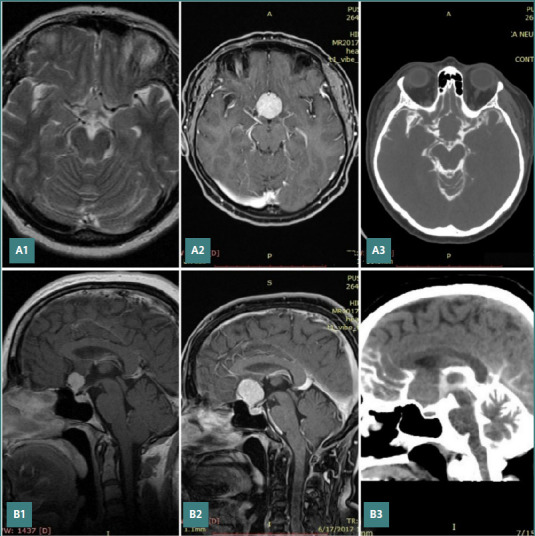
MRI and CT cisternography images depicting the relation between a meningioma arising in the suprasellar region and the subarachnoid cisterns and how tumor growth displaces the arachnoid structures. The patient (a 53-year-old woman) first presented to our service in 2013 with headaches and underwent an MRI. A1, B1, the initial MRI showed a small meningioma originating at the level of the tuberculum sellae. She refused any surgical intervention. In 2017, she presented with visual disturbances. A2, B2, the MRI reveals tumor growth. CT cisternography shows the preservation of the arachnoid layers and displacement of the cisterns, confirming the mentioned concept of tumor development. A3, B3, CT cisternography shows the preservation of the arachnoid layers and displacement of the cisterns, confirming the mentioned concept of tumor development.
